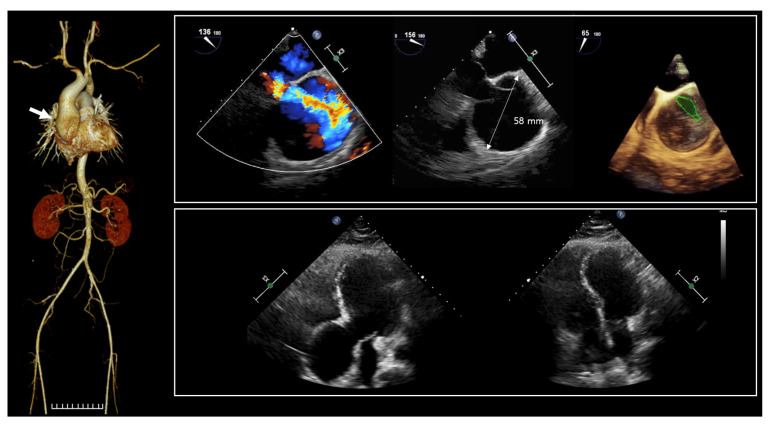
Computed tomography scan of the chest aorta revealed aneurysmal dilatation of about 5.8 cm from the aortic annulus to the proximal ascending aorta (arrow). Color doppler echocardiography of aortic root aneurysm; red and blue represents flow towards and away from the transducer, respectively. A 3-dimensional transesophageal echocardiogram shows aortic root aneurysm and bicuspid AV (sinus of Valsalva = 58 mm) (upper panel). Bicuspid AV is represented by green outline. Transthoracic echocardiography at present admission showed dilatation of aortic root and RV dilatation (lower panel).
